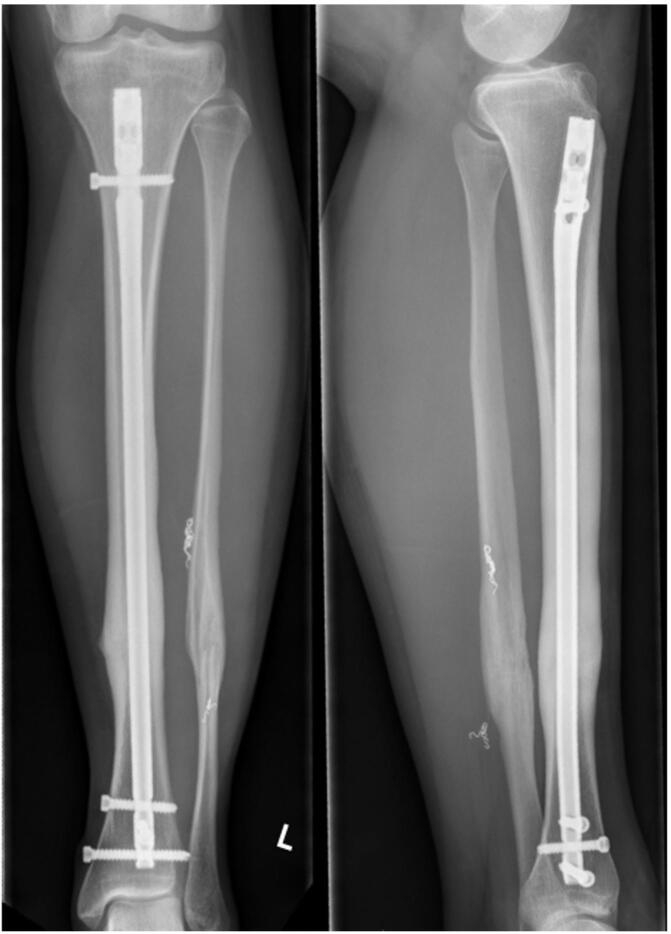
Follow up X-ray control one year following injury. Uneventful bony consolidation of the tibial and fibular fracture.
